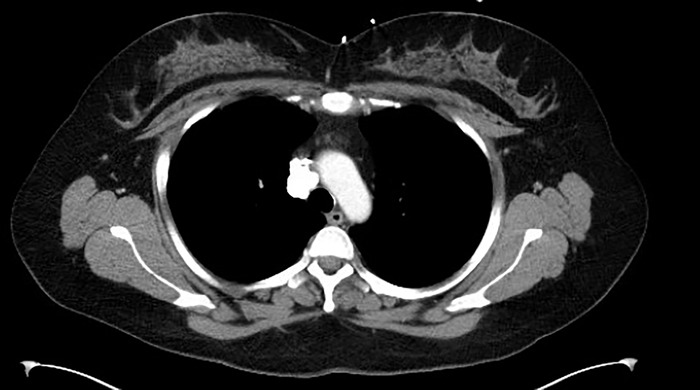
Computed tomography scan shows a large, calcified mass in the right paratracheal area.
